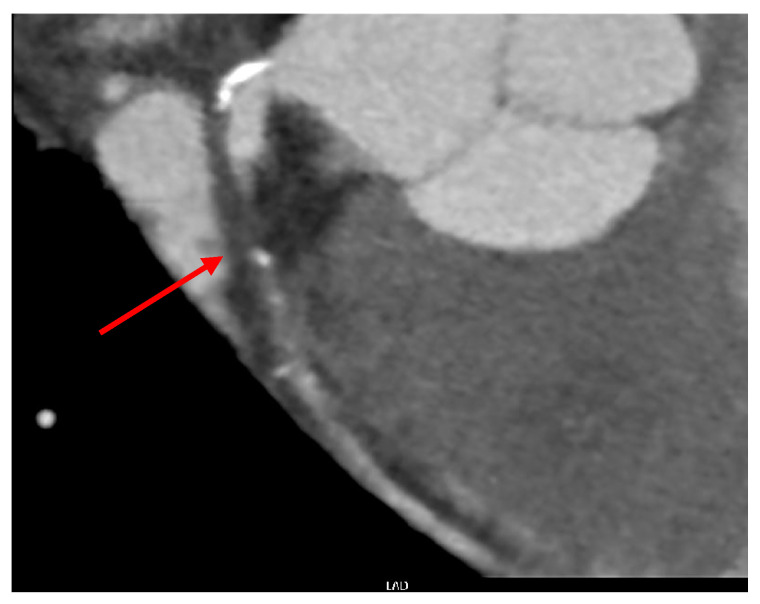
Near total occlusion of the left main descending artery (Arrow) indicated on CT heart angio and calcium score.
